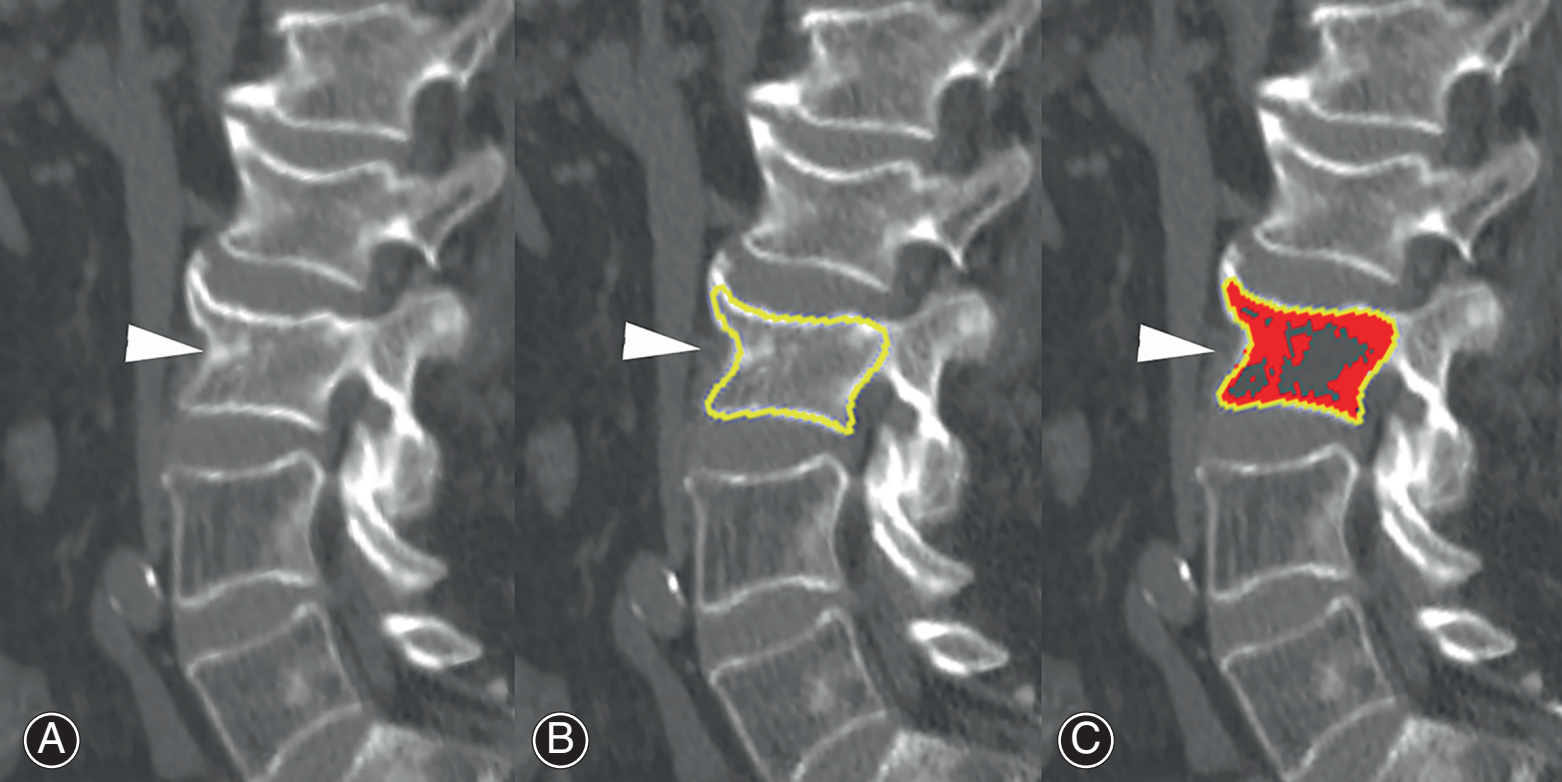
Fracture union assessed by ImageJ after rhPTH treatment. The original sagittal CT image of L2 (A, arrowhead). The region of interest is drawn according to the L2 outline (B, yellow line). Bone bridge connecting the upper and lower endplates is detected by setting the CT values of 200–1000 HU (C, red color region), indicating bone healing. HU, Hounsfield unit
